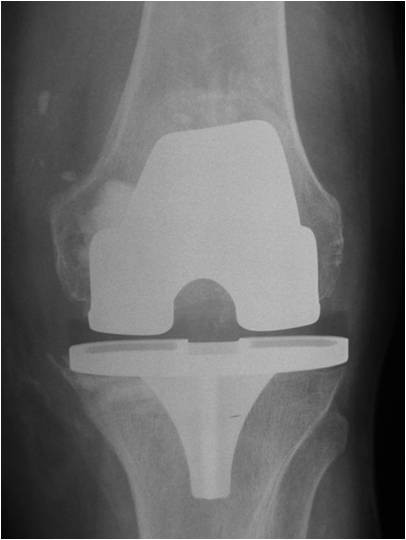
AP radiograph of the knee demonstrating the area of calcium phosphate bone cementage in the medial femoral and medial tibial condyles.
